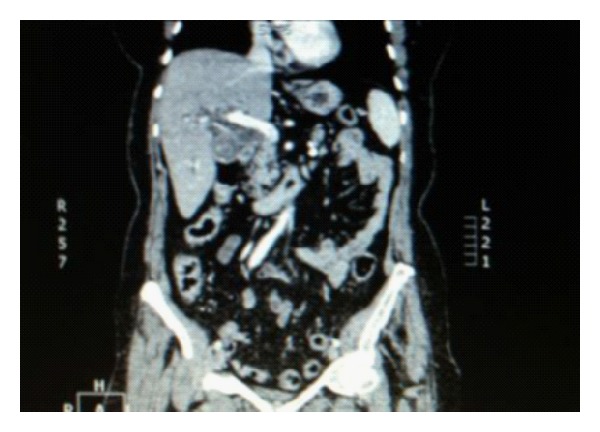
Abdominal computed tomography confirming the ultrasound findings and excluding malignancy in the porta hepatis area, in the liver, and in the pancreas.
